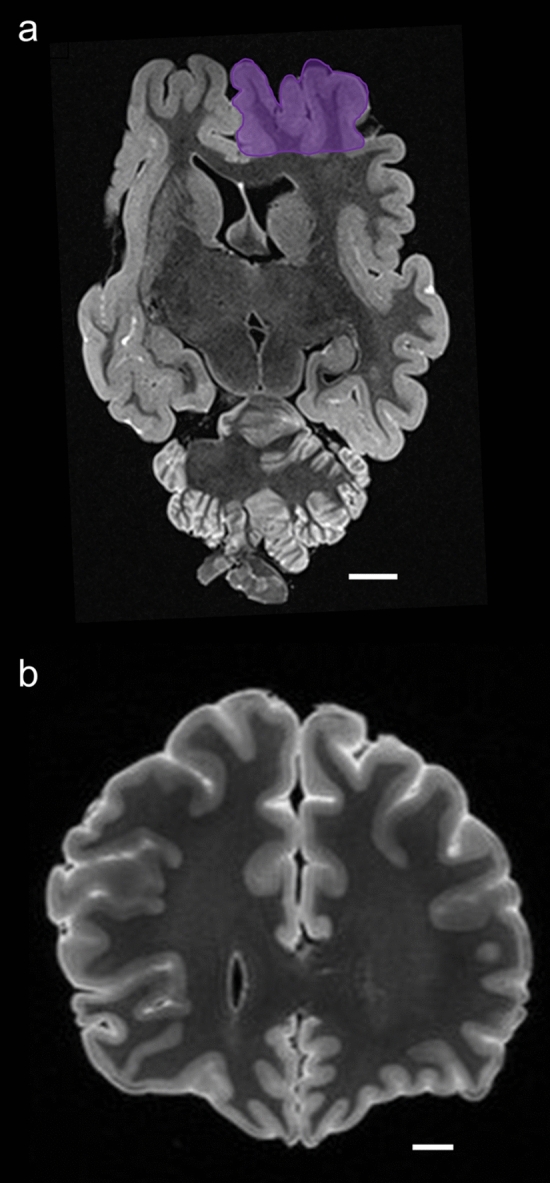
Magnetic resonance imaging (MRI) coronal scans of a muskox and a human brain. a Male muskox brain ex vivo, before sampling; the right prefrontal cortex is shaded in purple. Deformation and damage to the cerebellum occurred postmortem. Image obtained by coronal T2-weighted turbo spin-echo images obtained from an ex vivo old male muskox brain at 0.57 mm in-plane resolution and 1 mm slice thickness using a 7 T MRI scanner. b Human brain with a history of TBI, frame taken through the human prefrontal cortex at the level of the corpus callosum. Image obtained by ex vivo coronal 3D T2-weighted fluid attenuated inversion recovery (FLAIR) turbo spin-echo image acquired at 3 T at 0.63 mm isotropic resolution. Scale bar = 1 cm
